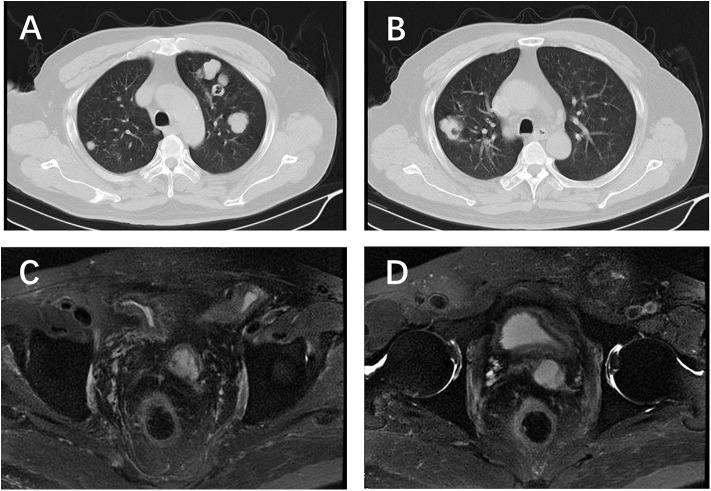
Chest CT and pelvic MRI before etoposide chemotherapy. (A,B) Multiple nodules located in both sides of the lung; (C,D) no visible recurrence shown on the pelvic MRI.
